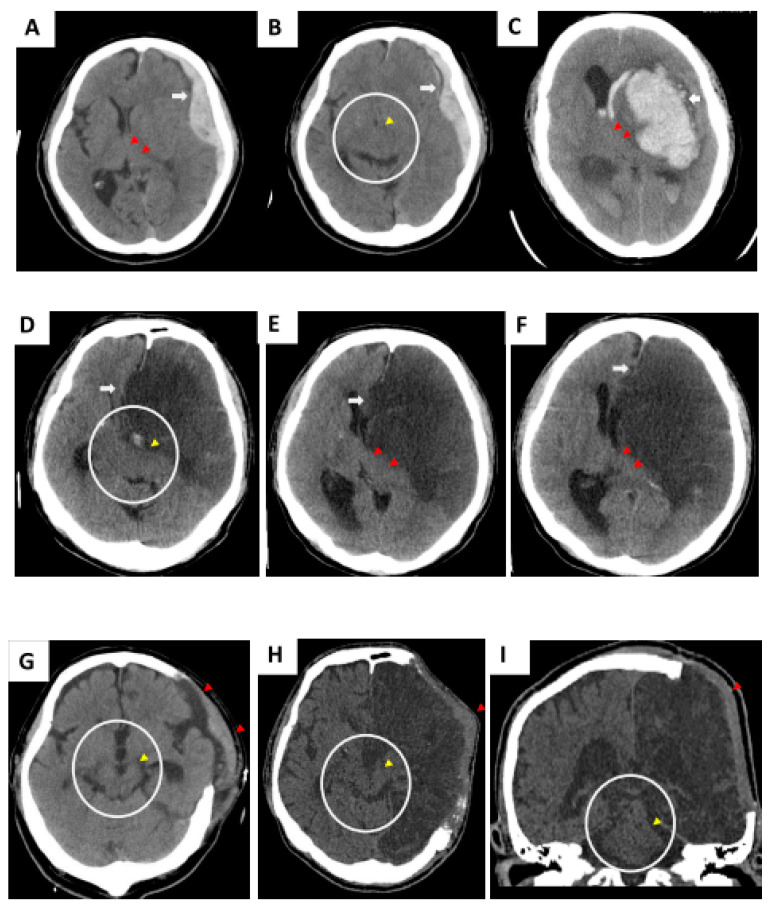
Initial computed tomography (CT) scan showing compromised brainstem due to acute subdural hematoma (SDH) (A,B, case 1), hemorrhagic stroke (C, case 2), and malignant cerebral infarction (D–F, case 3). Alteration of brainstem shape was caused by intracranial mass effect. Wide decompressive craniectomy (DC) should be performed immediately to create extensive volume, which accommodates postoperative brain swelling. (G–I) Brainstem shape was morphologically regained.
